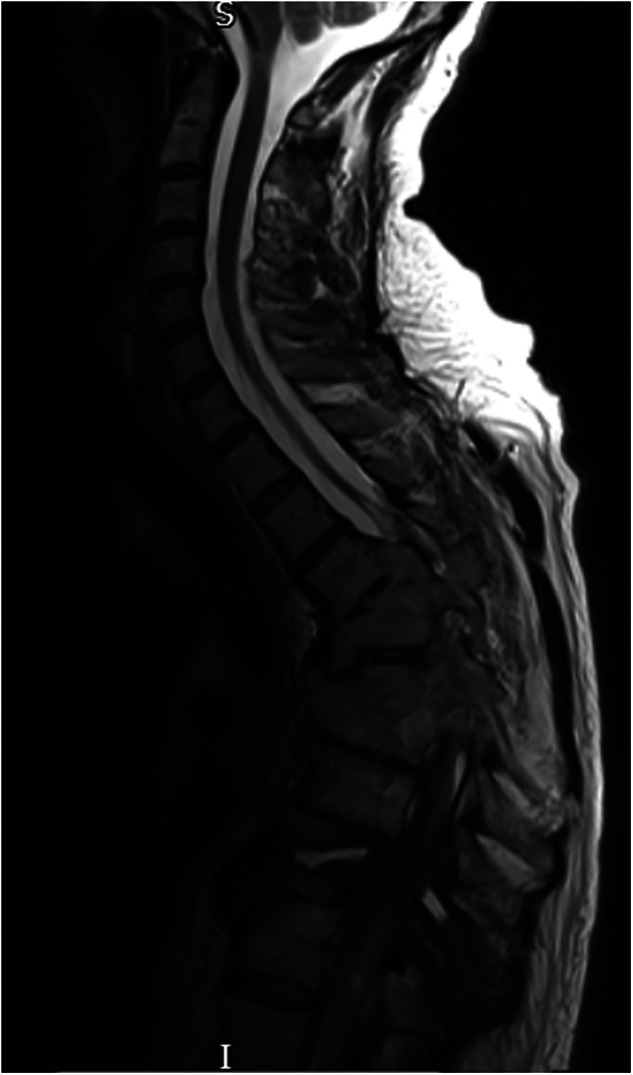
Sagittal view of the cervical and thoracic spine CT: there is evidence of chronic injury and post-traumatic fusion of T3, T4, T5, T6, T7 and T8. At the inferior aspect of this fused segment there is destruction of the T11 vertebral body with a fluid filled cleft extending through all three columns consistent with Charcot spine formation. There is no local marrow oedema within the T12, T10 and T9 vertebral bodies. There is severe cord compression at the level of T10 Charcot pseudoarthrosis and hyperintense signal within the distal cord and conus.
